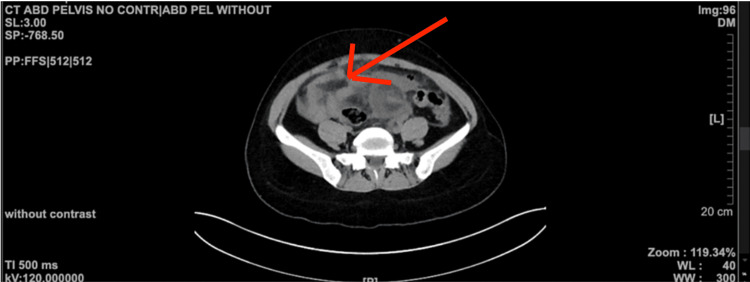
CT scan of the abdomen and pelvis conveying the abscess (red arrow) in the lower abdomen prior to pigtail insertion CT: computed tomography
